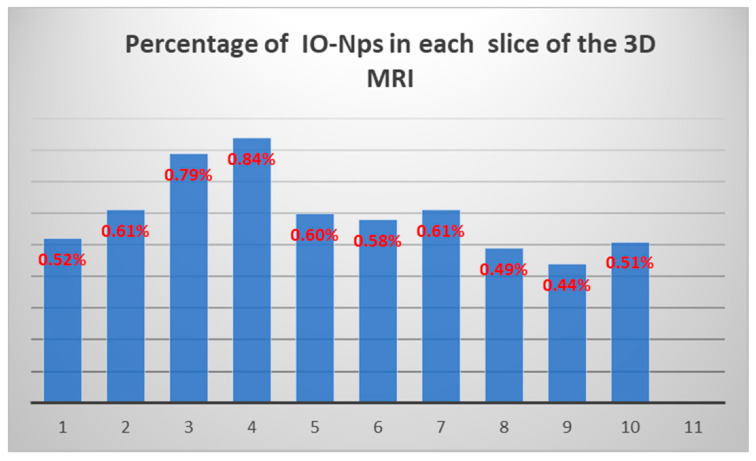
Percentage of IO-NPs of 10 slices from 3D MRI image.
